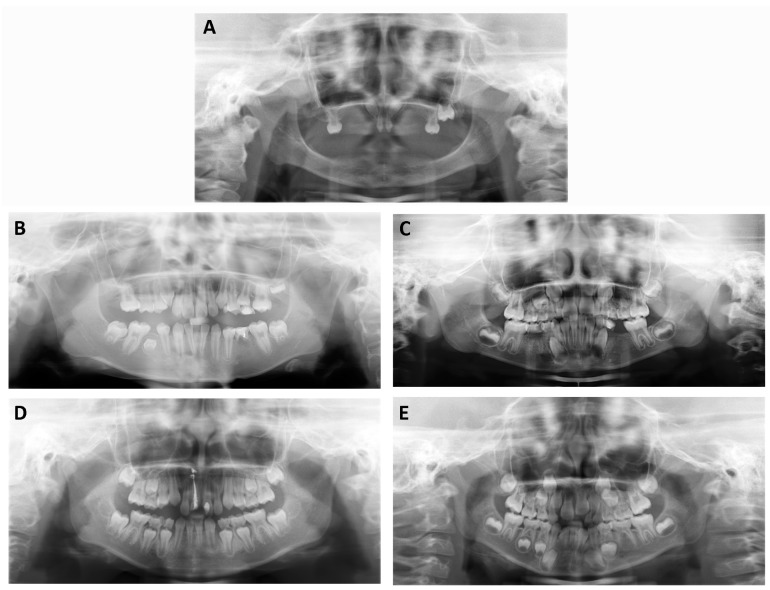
Panoramic radiograph of the probands of X-linked hypohidrotic ectodermal dysplasia (XLHED) Family 2 (A); non-syndromic tooth agenesis (NSTA) Family 1 (B); NSTA Family 2 (C); NSTA Family 3 (D); and NSTA Family 4 (E). They were missing 23, 4, 9, 2, and 6 permanent teeth, respectively. Because the proband of XLHED1 was too young to cooperate, we were not able to assess the missing teeth of the patient by panoramic radiograph. For the uncompressed Figure 1 file, please download the Supplementary Materials.
